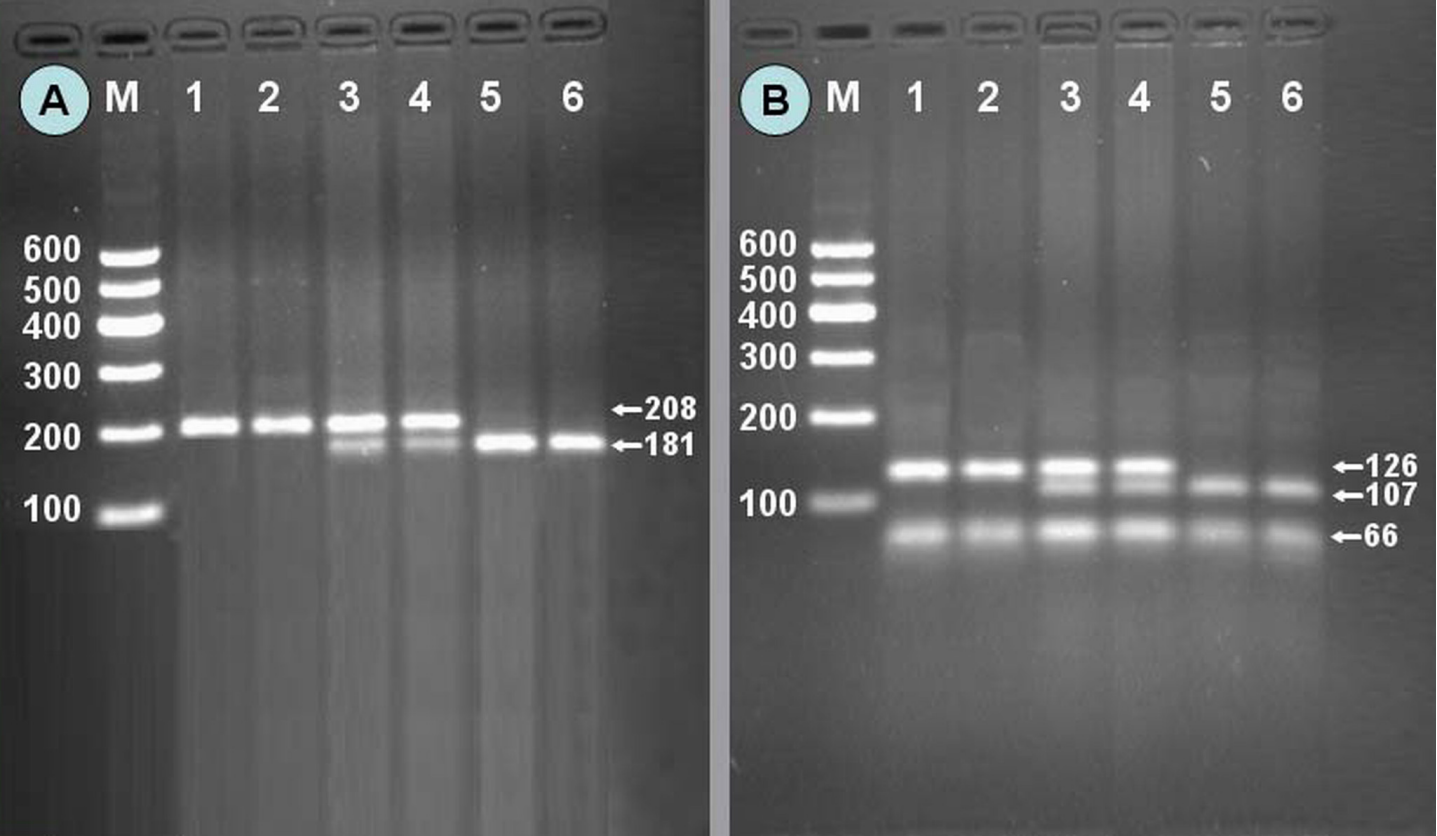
Genotyping of the *GALNT2 *rs2144300 and rs4846914 polymorphisms. (A) *GALNT2 *rs2144300. Lane M, 100 bp marker ladder; lanes 1 and 2, CC genotype (208 bp); lanes 3 and 4, CT genotype (208-, 181- and 27-bp); and lanes 5 and 6, TT genotype (181- and 27-bp). (B) *GALNT2 *rs4846914. Lane M, 100 bp marker ladder; lanes 1 and 2, AA genotype (126- and 66-bp); lanes 3 and 4, GA genotype (126-, 107-, 66- and 19-bp); and lanes 5 and 6, GG genotype (107-, 66- and 19-bp). The 27- and 19-bp fragments were invisible in the gel owing to their fast migration speed.
